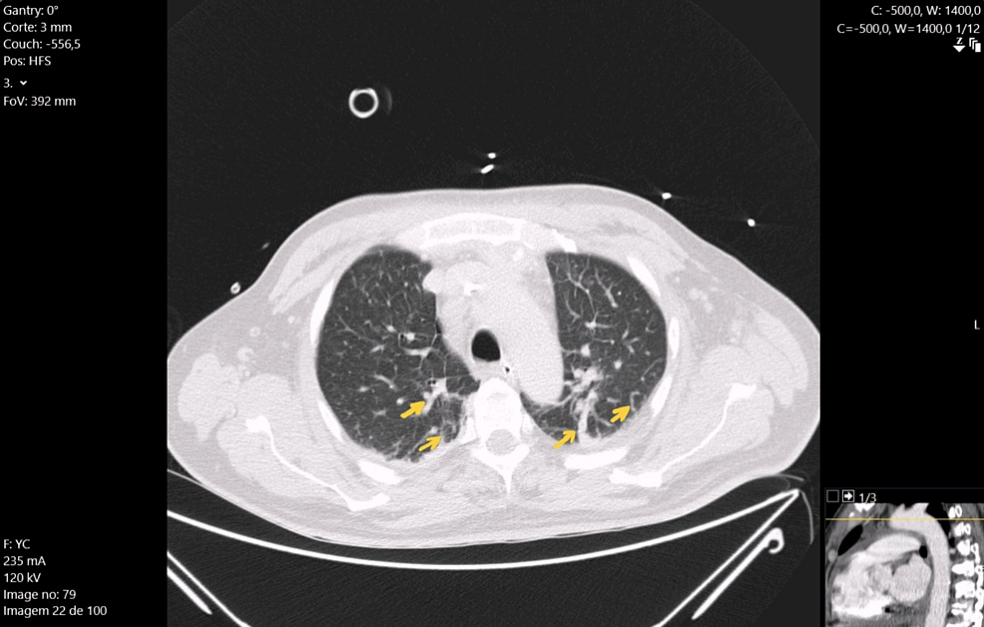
Thoracic CT scan revealing a bilateral peripheral linear image suggestive of vascular dilatations (yellow arrows)
